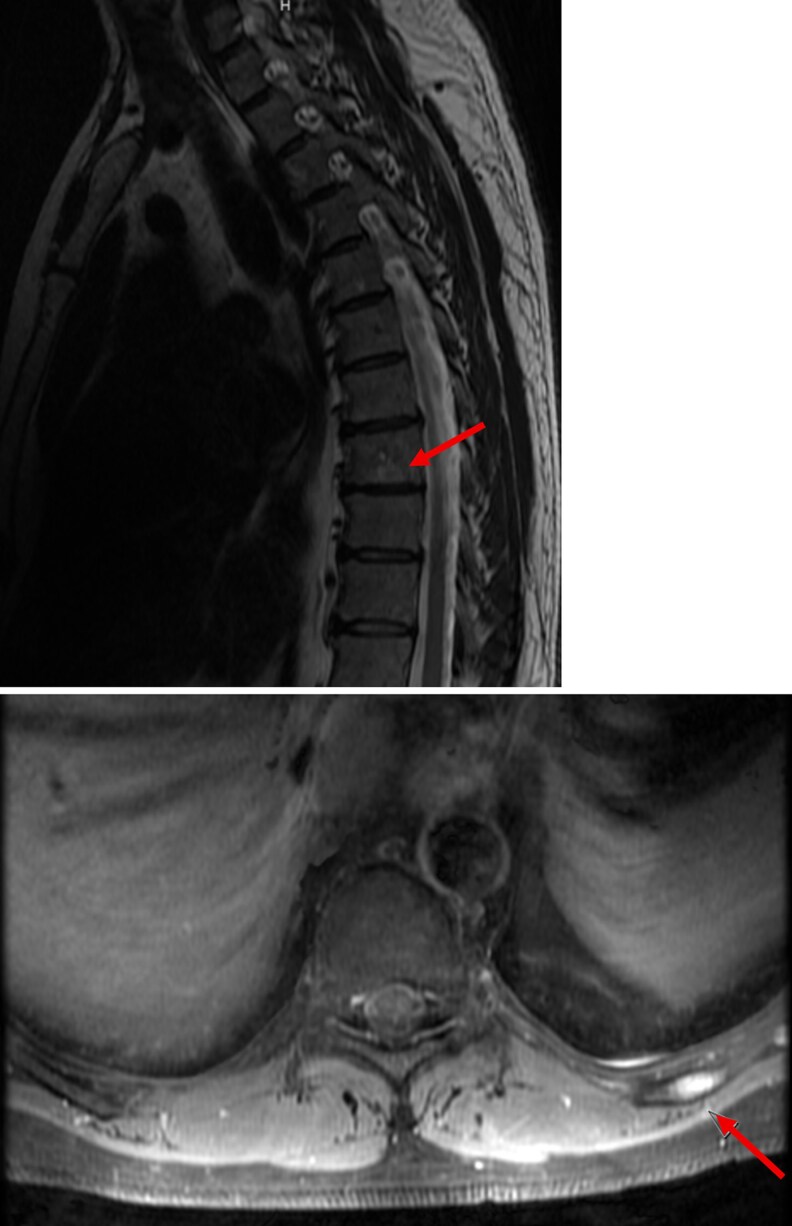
Case #2 MRI thoracic spine. (Top) 10-mm T1 hypointense lesion with mild enhancement in the T8 vertebral body. (Bottom) Left rib with expansile enhancement.
